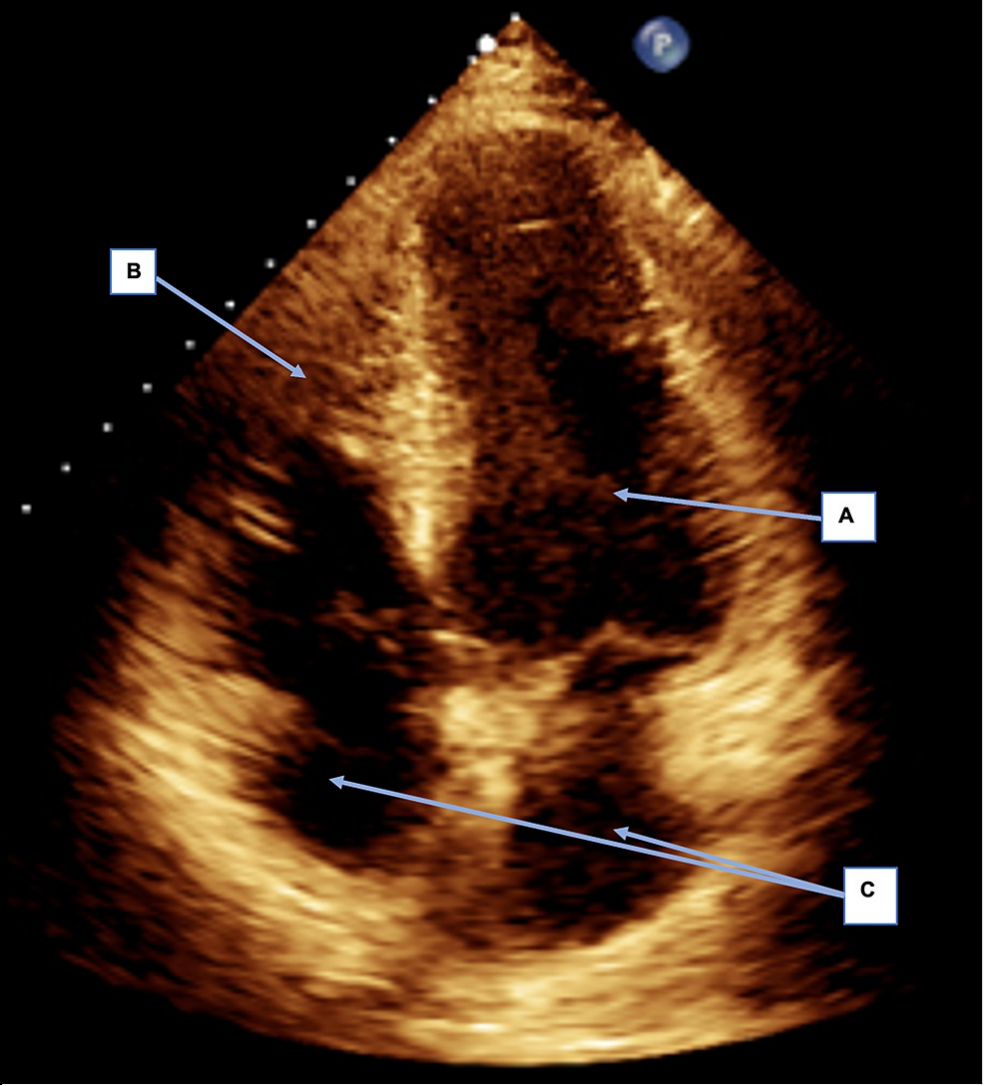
Pre-treatment TTE: Apical 4 chamber (A4C) view in diastole, showing reduced LV systolic function. A: Left ventricle visually appears dilated in this still image, suggestive of impaired function in diastole;
